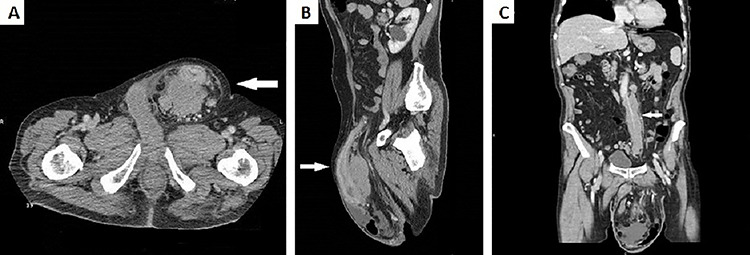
(A) CT scan of the abdomen demonstrated a huge left inguinal hernia containing distal descending colon and sigma (white arrow); (B) CT sagittal scan of the abdomen demonstrated sigma walls thickening inside left inguinal region (white arrow); (C) CT scan of the abdomen showed a bulky adenopathy encircling IMA just below its origin without vessel infiltration (white arrow).
